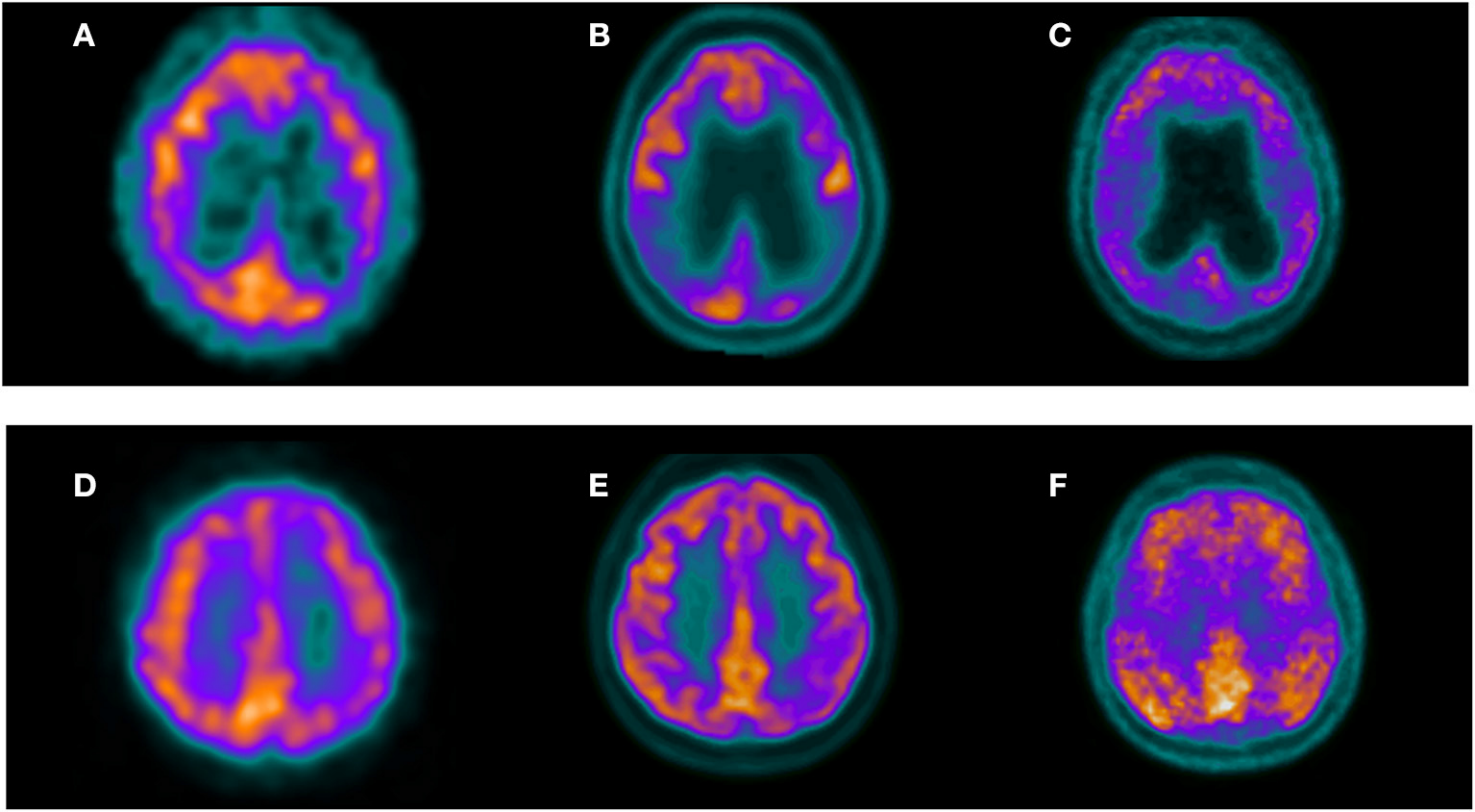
Three selected transaxial slices of the perfusion SPECT, ^18^F-FDG PET, and ^11^C-PIB PET of two patients referred for clinical evaluation of cognitive impairment. Superior row corresponds to a 63-year-old female with a mild cognitive impairment. MMSE was 27. Both perfusion SPECT (A) and ^18^F-FDG PET (B) showed hypoperfusion/hypometabolism in the bilateral parietal cortex with a typical pattern of AD. Amyloid PET in this patient (C) showed significant cortical amyloid deposits. Inferior row shows a 55-year-old female referred for evaluation of probable AD. Both perfusion SPECT (D) and ^18^F-FDG PET (E) showed a left posterior parietal hypoperfusion/hypometabolism suggestive of AD. ^11^C-PIB PET in this patient (F) confirmed cortical amyloid deposits.
